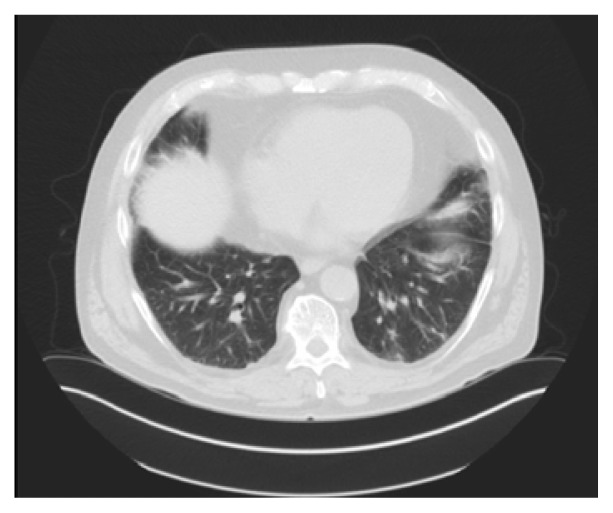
Comparative CT of the chest showed resolution of the left pleural effusion.
